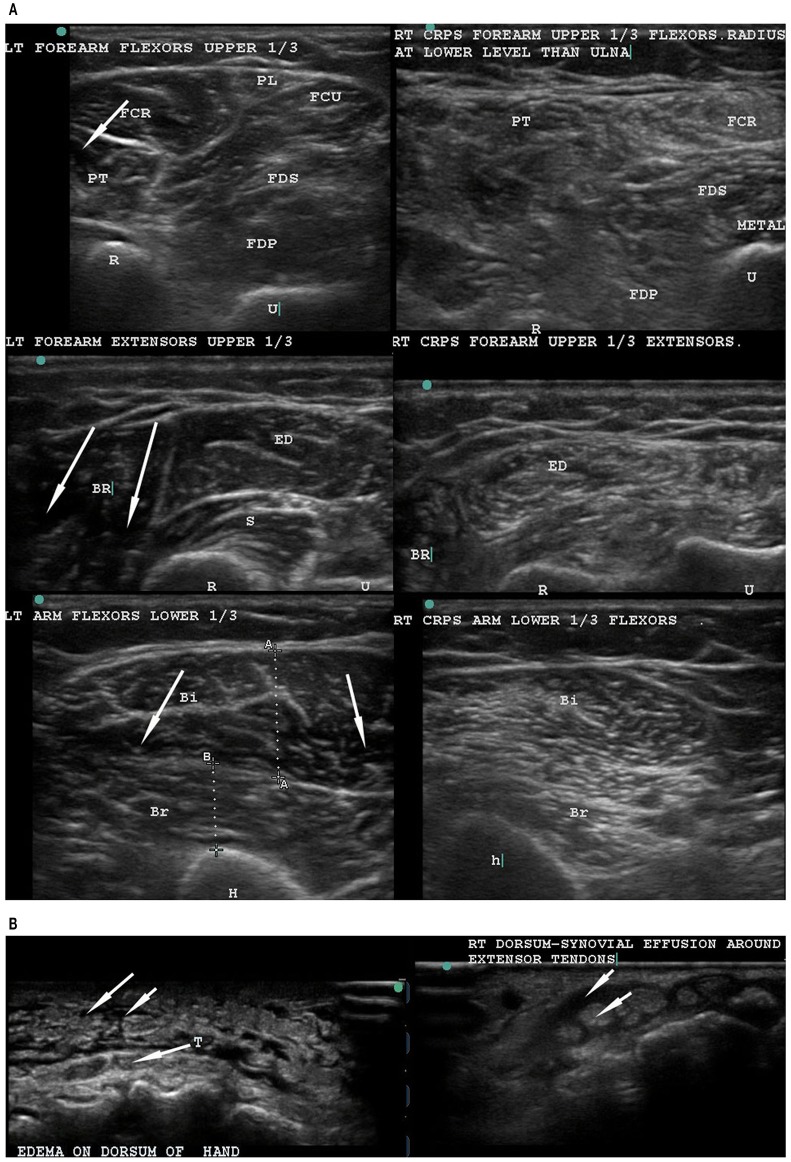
Musculoskeletal ultrasonography to compare the normal left arm with the CRPS-affected right arm. (A) Musculoskeletal ultrasound of the normal left forearm (left) and the CRPS-affected right forearm (right) of a single patient. The normal left extremity muscles have well-defined outlines, and the muscle is hypoechoic (dark spaces, see arrows) with bright curvilinear echoes. In contrast, the CRPS-affected right arm and forearm muscles show general hyperechogenecity (as indicated by a snowstorm-like appearance not seen in normal muscle), with loss of the dark spaces as well as muscle outlines. (B) Ultrasonography of the dorsum of hand in a non-CRPS patient (left) and a CRPS patient (right). In the non-CRPS patient, the edema is subcutaneous (left, upper arrows) and superficial to the tendons (T) (arrow from T). In the CRPS-affected hand, a peritendinous tenosynovial effusion (right, upper arrow) surrounds the tendon (right, lower arrow). Note the normal narrow subcutaneous space without any fluid accumulation. T, tendon.
